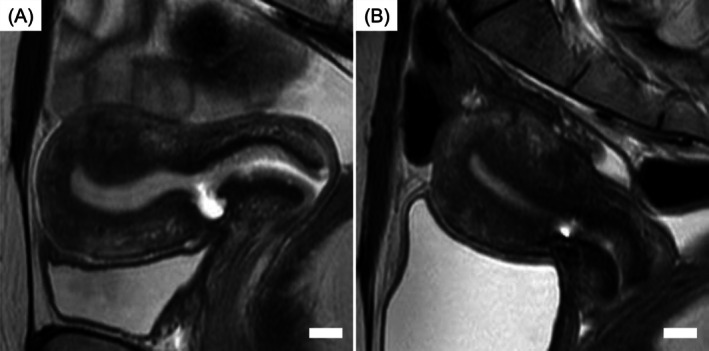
T2‐weighted sagittal MRI findings of the uterus before pregnancy. (A) MRI before cesarean scar repair. A cesarean scar niche measuring 8.1 mm in depth and 6.7 mm in width was observed. The RMT was 1.8 mm, and the cervical length measured 36.8 mm. (B) MRI after cesarean scar repair. The RMT increased to 6.5 mm, and the cervical length measured 28.3 mm. The white bars indicate 10 mm. MRI, magnetic resonance imaging; RMT, residual myometrial thickness.
